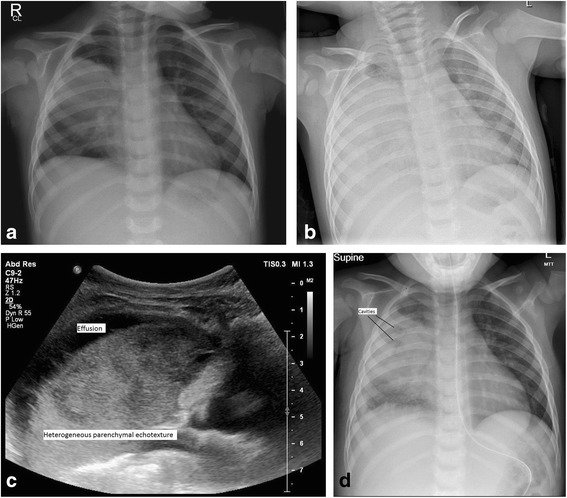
A 2-year-old child presenting with 4 days of fever, cough, and intermittent grunting respirations. Pleural fluid tested positive for pneumococcal antigen. a Initial plain chest radiograph showing right mid-zone airspace opacity, consistent with pneumonia. b Plain chest radiograph 24 h later revealing a large right-sided pleural effusion. c Lung ultrasonographic image taken immediately afterwards depicting the pleural effusion and heterogeneous parenchymal echo texture, consistent with an underlying necrotizing pneumonia. d Plain chest radiograph 4 days later following thoracoscopy and removal of chest drains demonstrates right upper zone cavities within the region identified previously by ultrasonography
